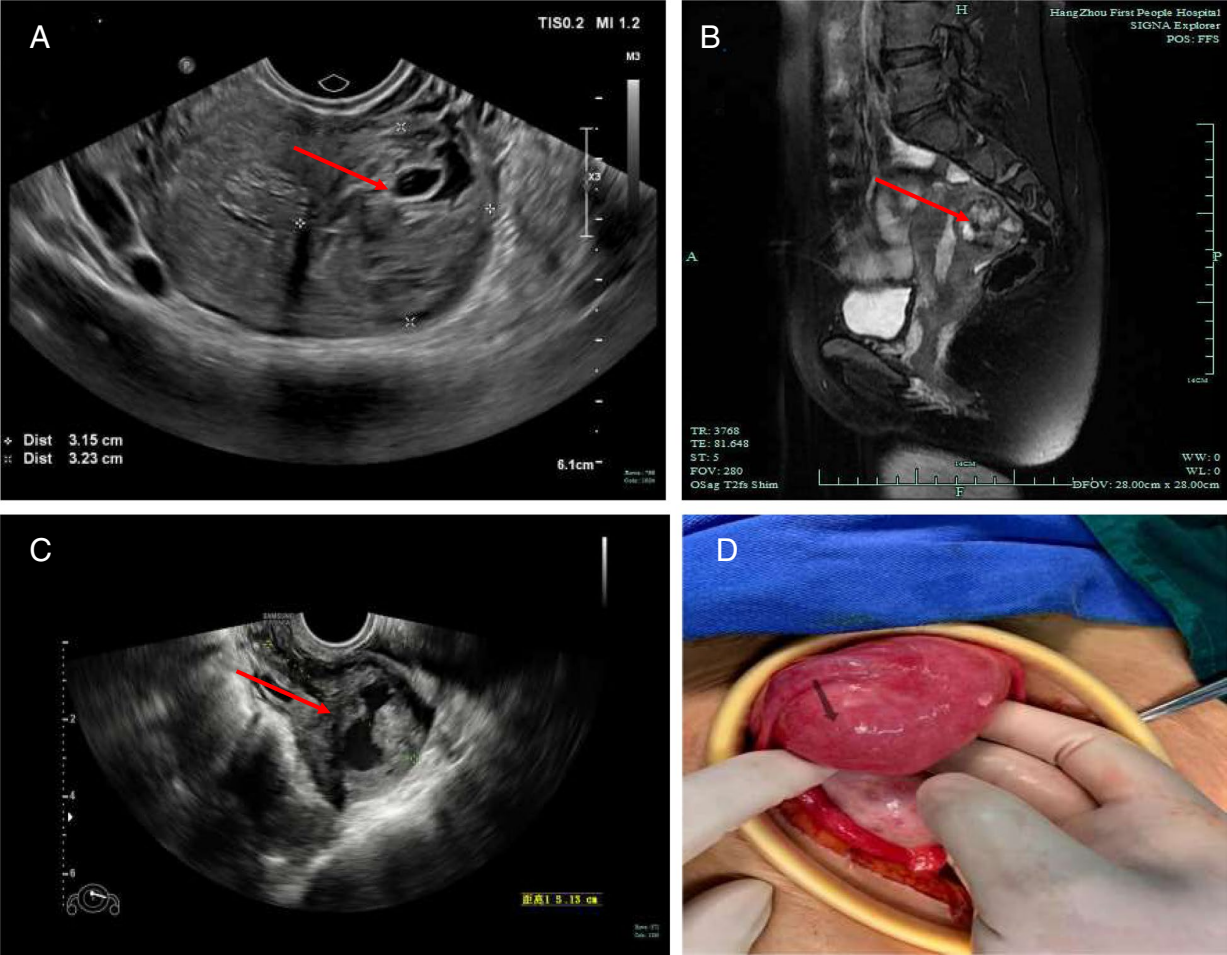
A Trans-vaginal ultrasound showed a left cornual heterogeneous echogenic mass about 4.3*3.2*3.2 cm. B Pelvis MRI scan showed a left cornual mass about 2.9*3.2*3.9 cm. C Trans-vaginal ultrasound showed a mixed-echo mass about 5.1*3.6*3.2 cm on the left uterine horn. D Open abdominal exploration revealed a round mass approximately 4 cm in diameter at the left uterine myometrium
